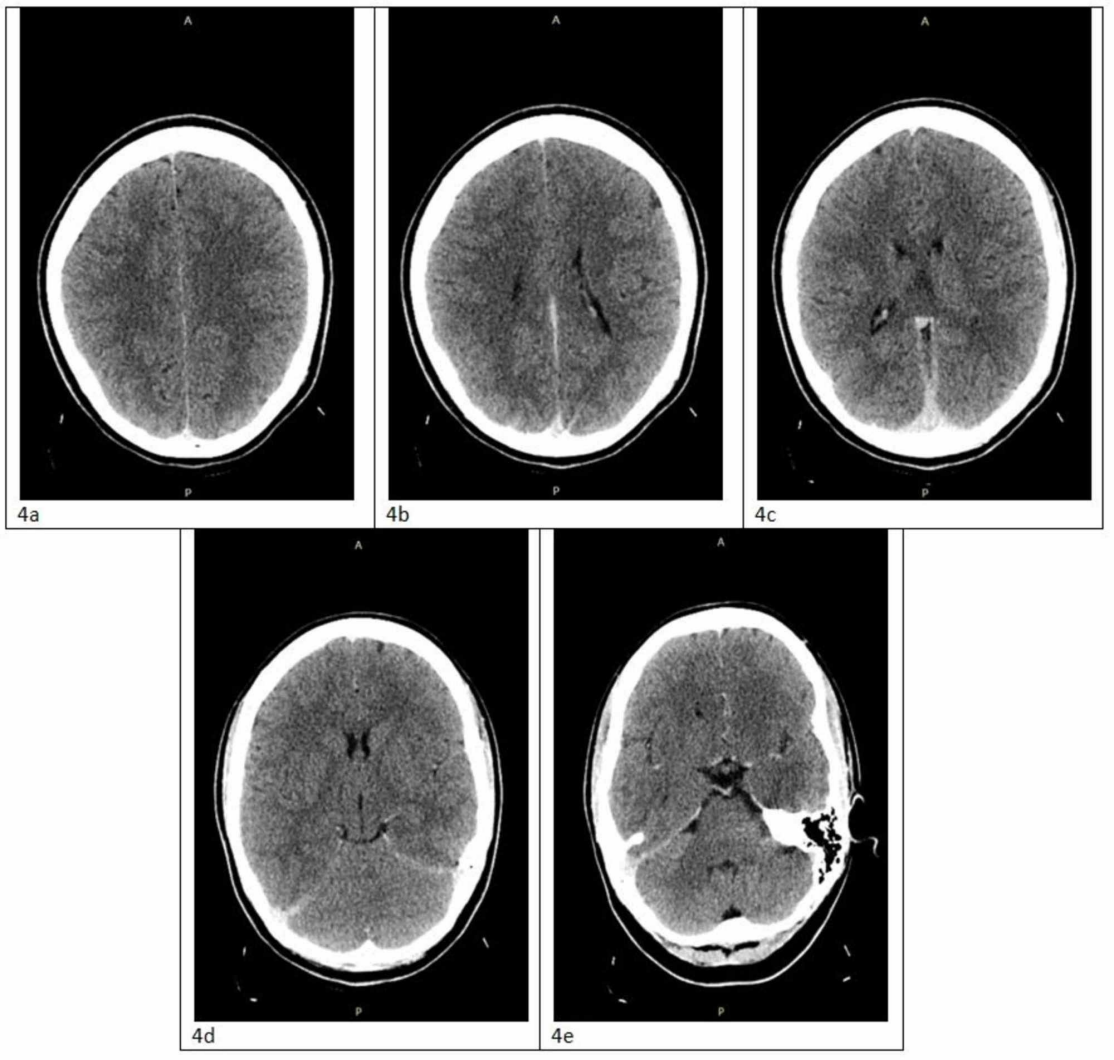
CT of the brain without contrast within normal limits (a-e) Views of the brain in different transverse planes (top to bottom)
